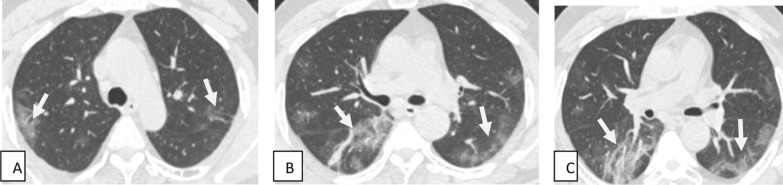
Male patient aged 54 years with cough, fever and myalgia. Axial (A–C) CT images show bilateral multifocal areas of ground-glass density (white arrows) seen at both lung fields with peripheral subpleural predominance (mild severity)
